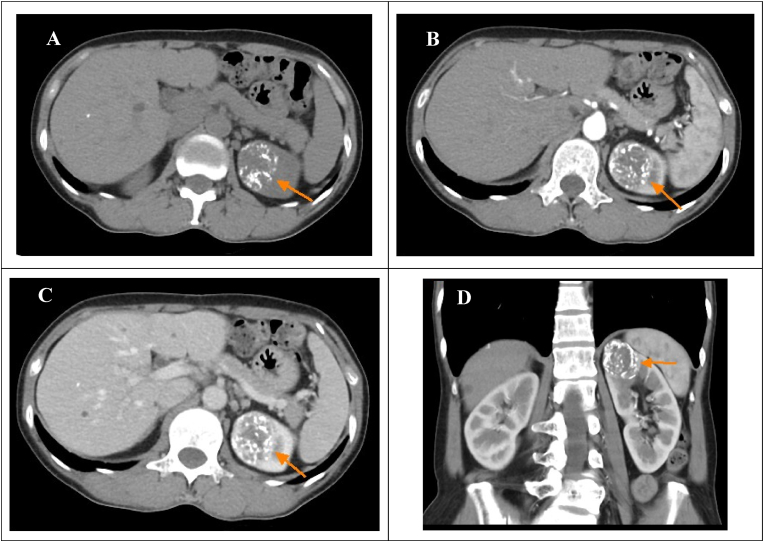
CT imaging of our cases (A) Precontrast CT showed a heterogenous renal tumor with calcification over the upper pole (B) Arterial phase (C) Venous phase (D) Coronal view.
